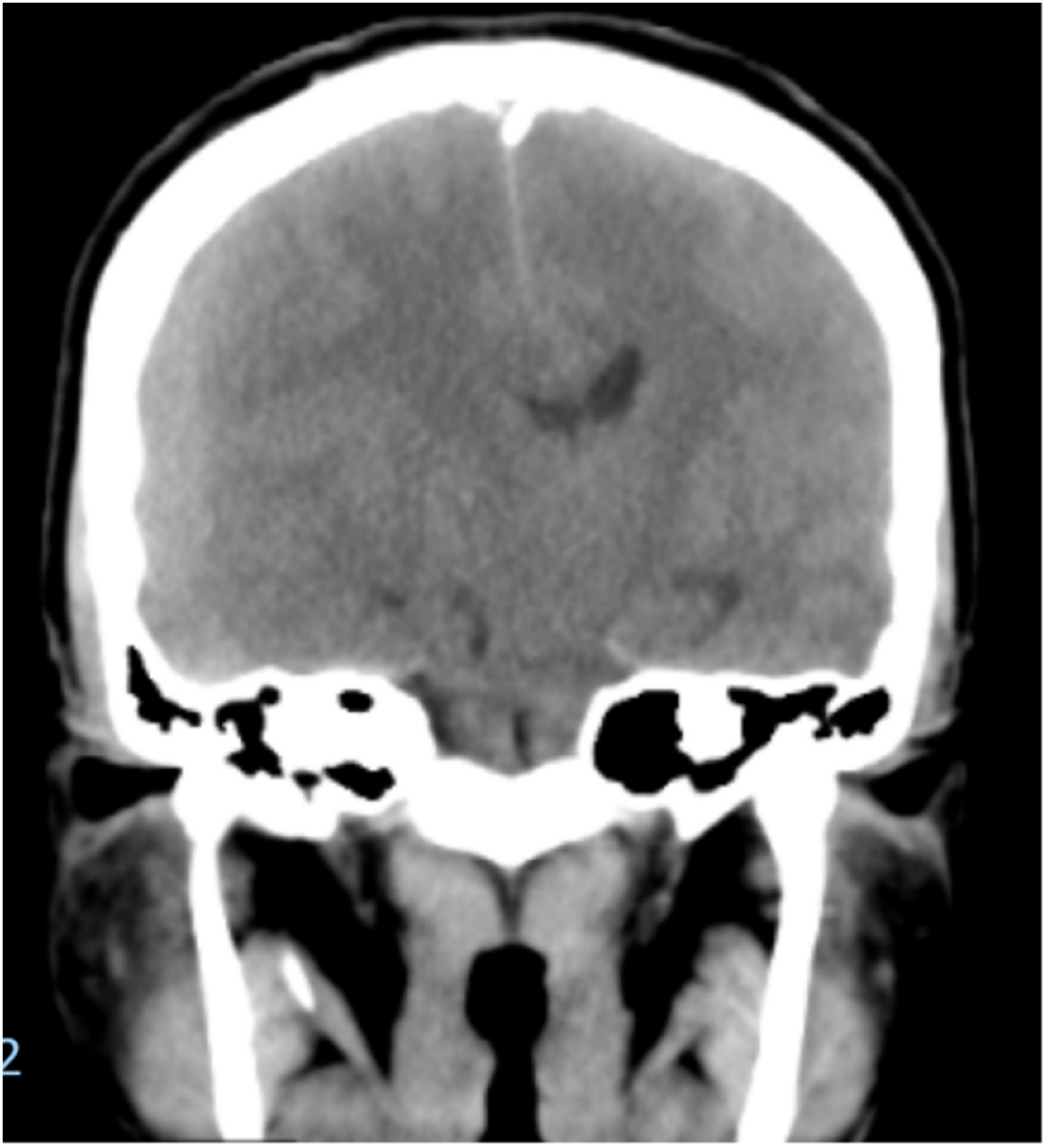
Computed tomography coronal image showing right subdural hematoma with midline shift.
